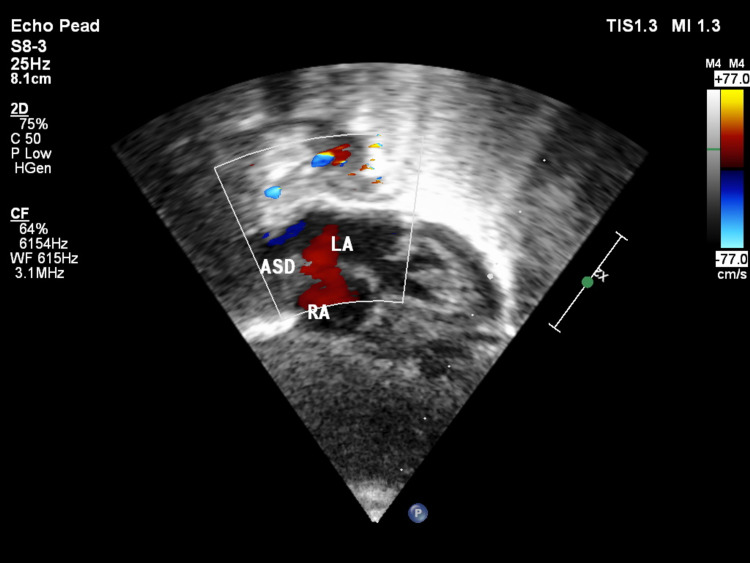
Two-dimensional echocardiogram image showing the atrial septal defect.
